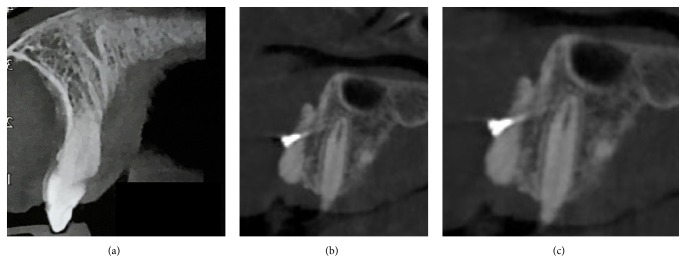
CCBCT images, before (a), immediately after (b), and after surgery (c).
